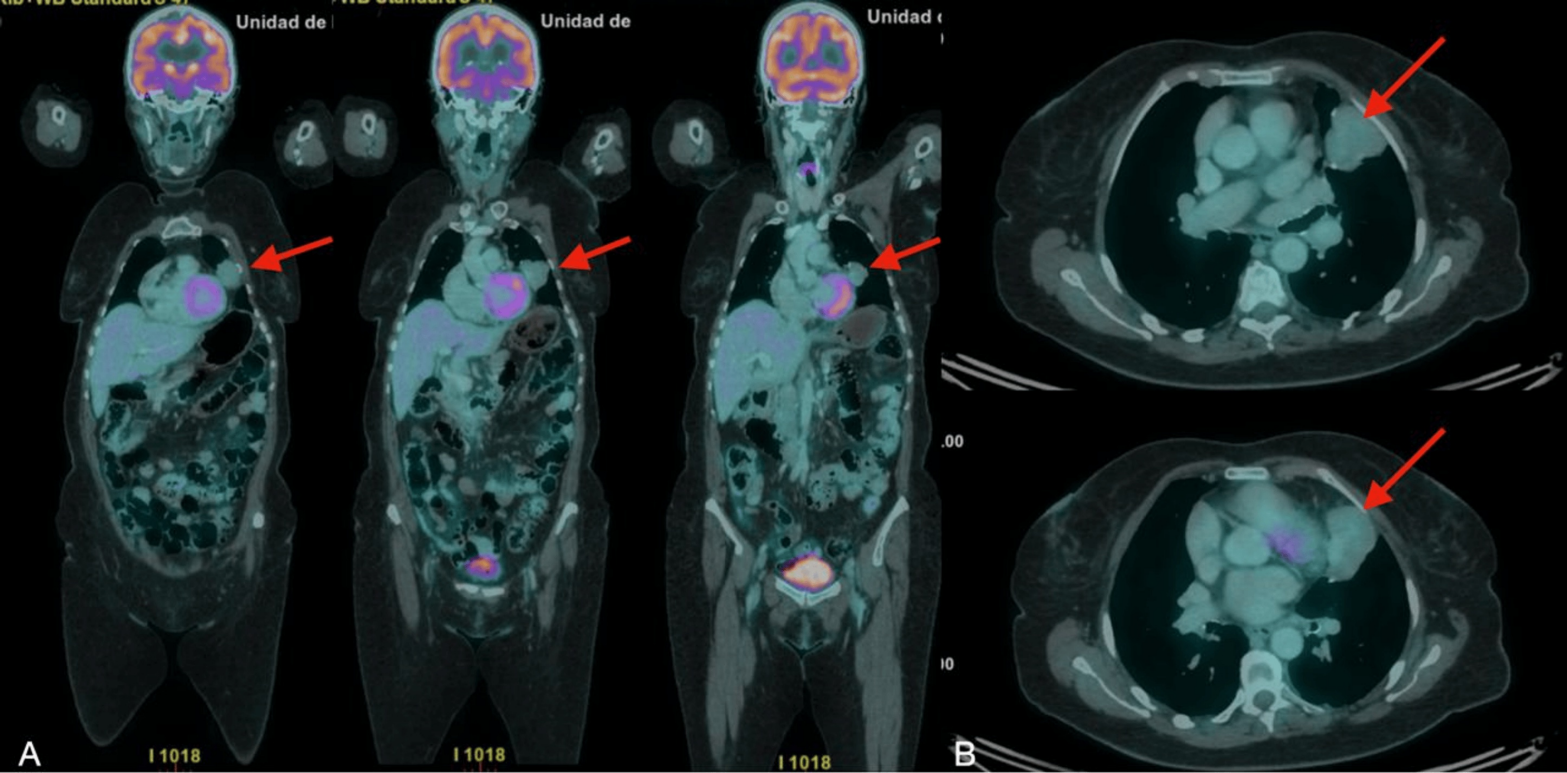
Positron emission tomography-computed tomography (PET-CT) findings of a recurrent solitary fibrous tumor. (A) Coronal fused 18F-fluorodeoxyglucose (18F-FDG) PET-CT images demonstrating a lobulated solid mass involving the lingular and anterior segment of the left upper lobe (red arrows), with low and heterogeneous FDG uptake.
